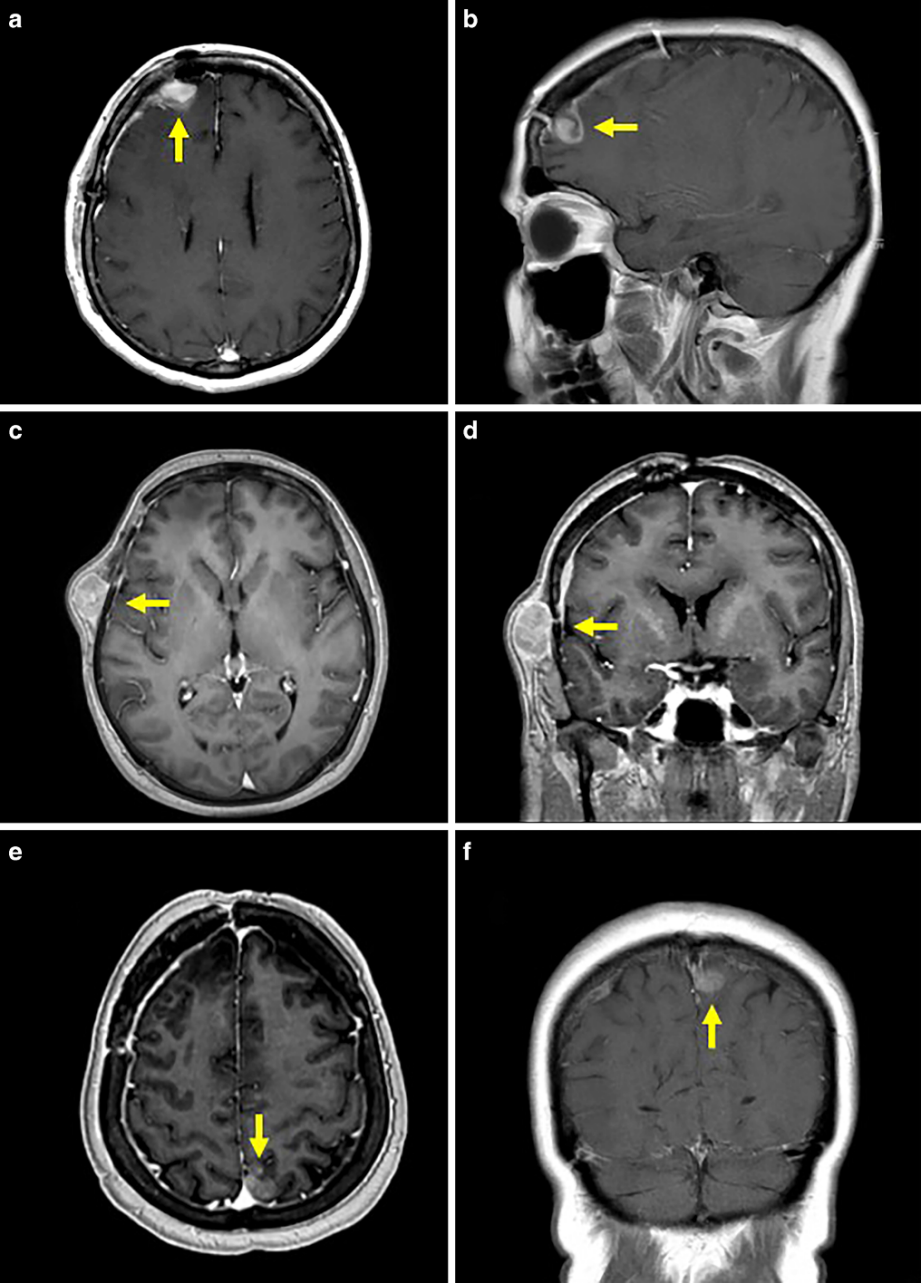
MRI scans of glioblastoma manifestations before systemic spread, in order of appearance: a, b initial manifestation with resection cavity localized in the right frontal lobe (arrow; postoperative imaging since preoperative imaging was not available); c, d first extracerebral manifestation in the left temporoparietal skull (arrow); e, f distant manifestation of the left occipital lobe (arrow)
